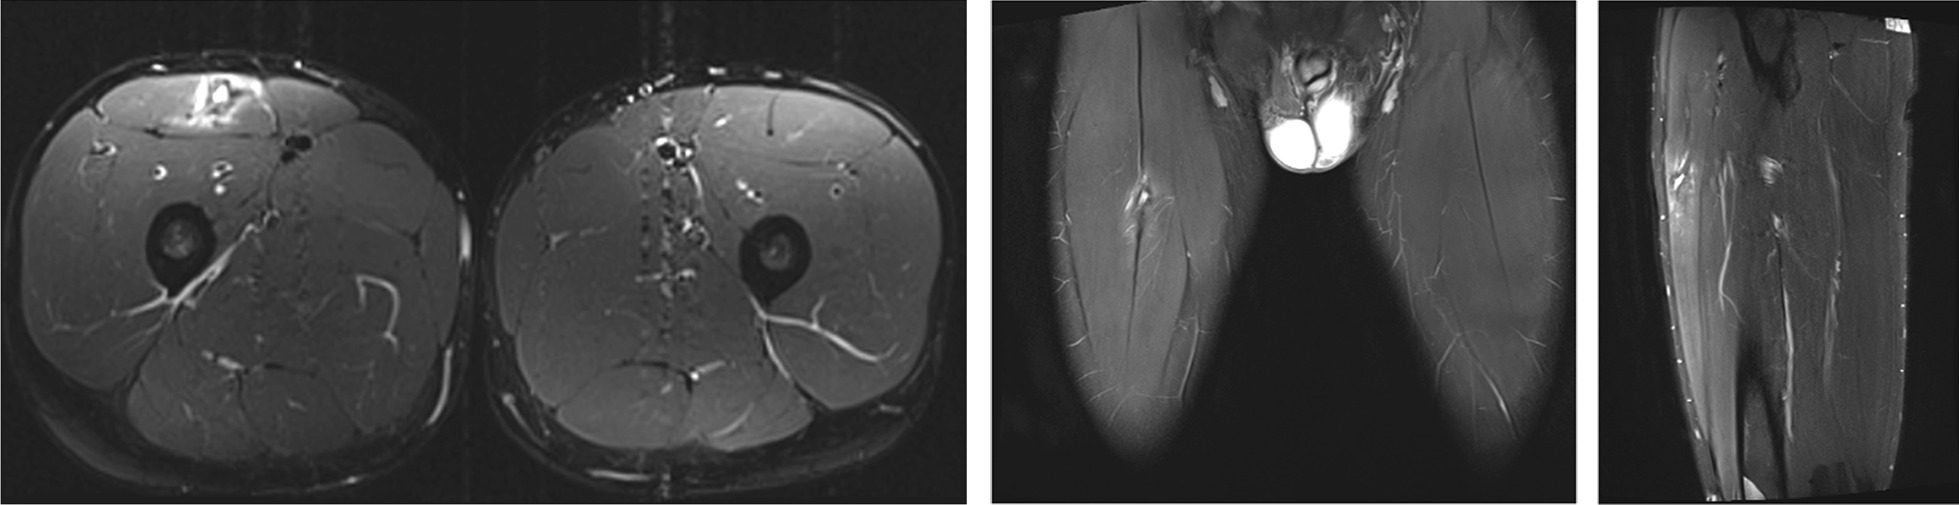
Coronal proton density fat saturated image that shows a persistent hematoma and a rupture in the central tendon. Distal to the hematoma the connective tissue shows scar changes and a mild loss of tendinous tension (MRI)
